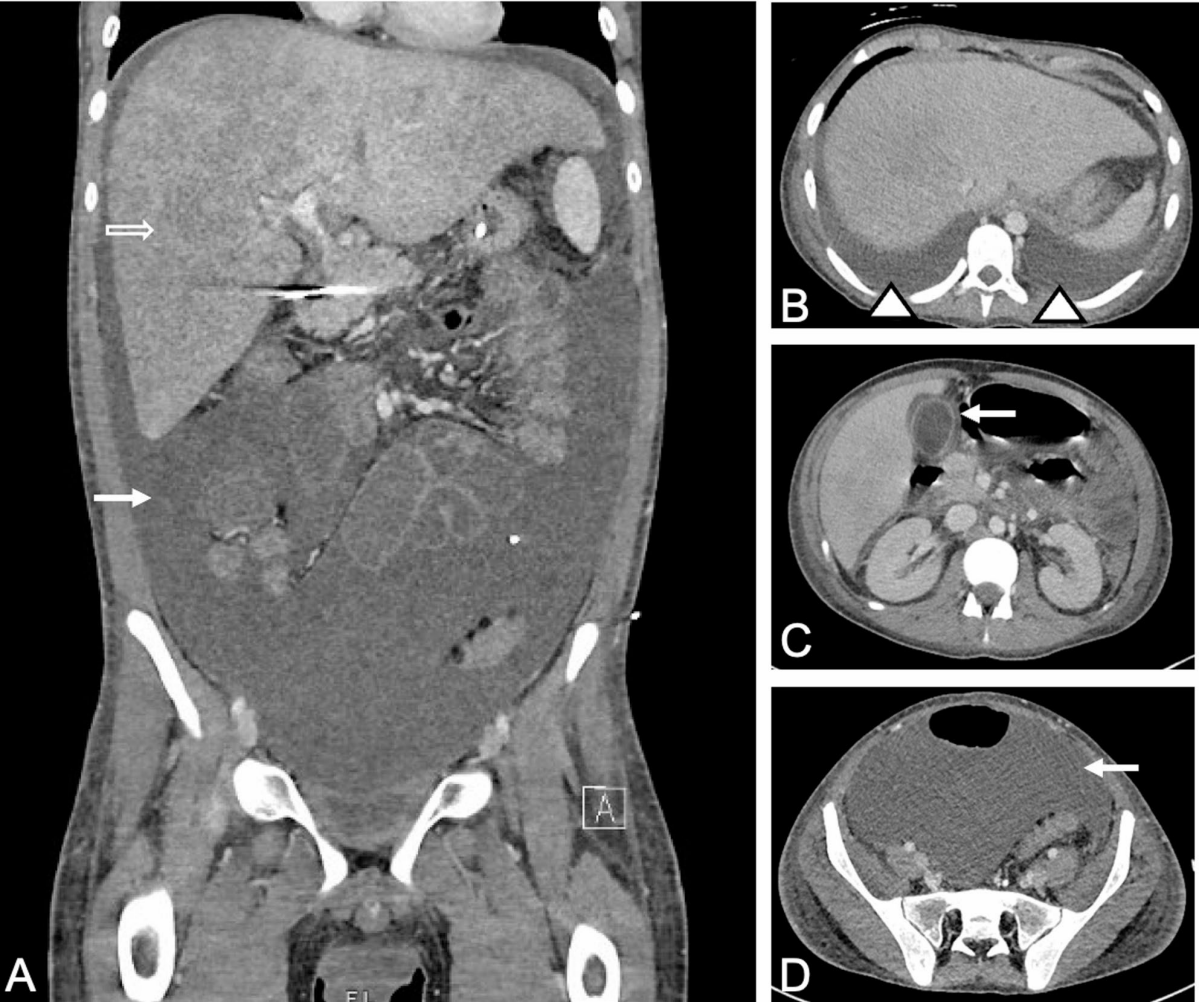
CRS in a 16-year-old young man with relapsed B-cell acute lymphoblastic leukemia, noted on a contrast-enhanced CT scan at 12 days following CAR T-cell therapy. A) Coronal contrast-enhanced CT demonstrates massive ascites (white arrow) and marked liver edema (open arrow). B) Axial scan through the lower chest shows bilateral pleural effusions (arrowheads). C) Axial CT through the upper abdomen demonstrates pericholecystic fluid (white arrow) and blunted enhancement of the kidneys, consistent with renal edema. D) Axial scan through the lower abdomen demonstrates extensive ascites (arrow). Findings are consistent with CRS-induced capillary leakage
